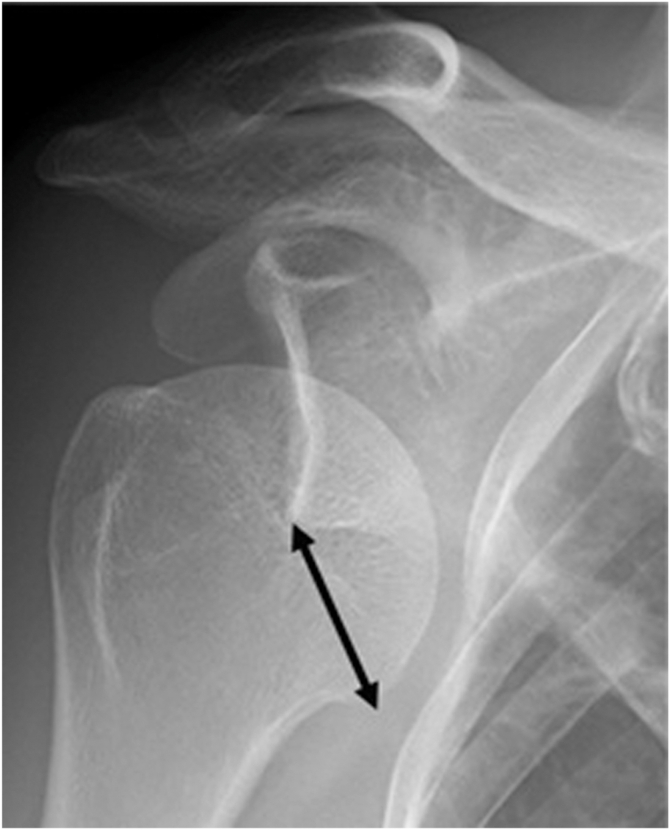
Measurement of dislocation distance on anteroposterior radiographs taken at the time of dislocation. The bidirectional arrow represents the distance between the inferior glenoid margin and the medial aspect of the humeral anatomical neck, defined as the dislocation distance.
